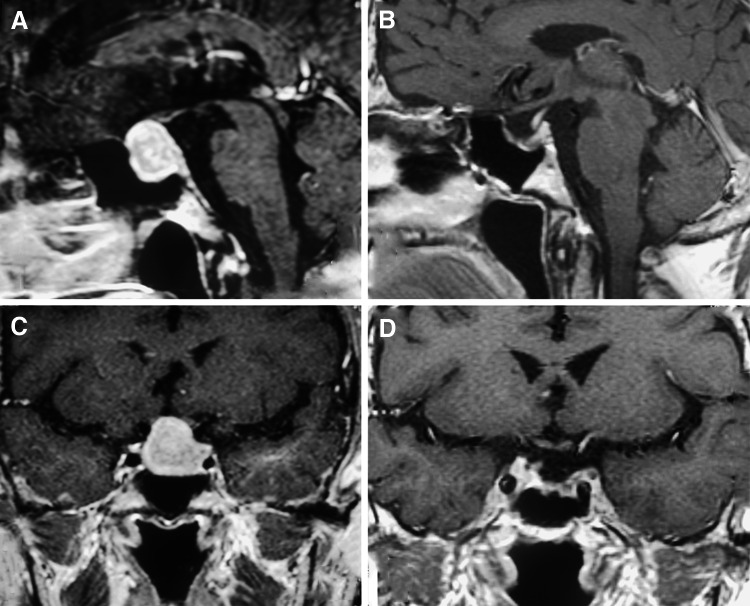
MRI images of one TSH adenoma. A, C Before surgery, B, D gross total resection after surgery
